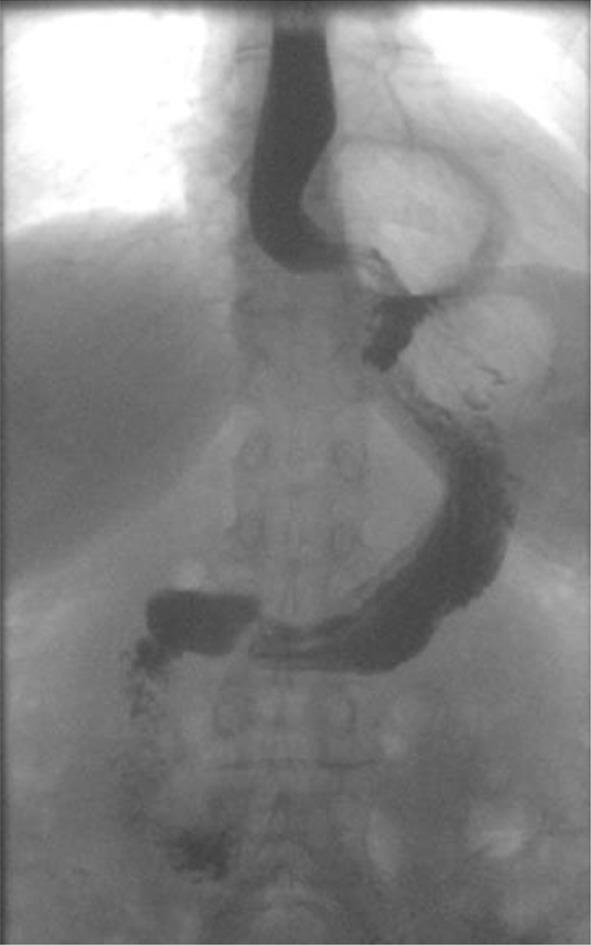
Barium X-ray film demonstrating reherniation
